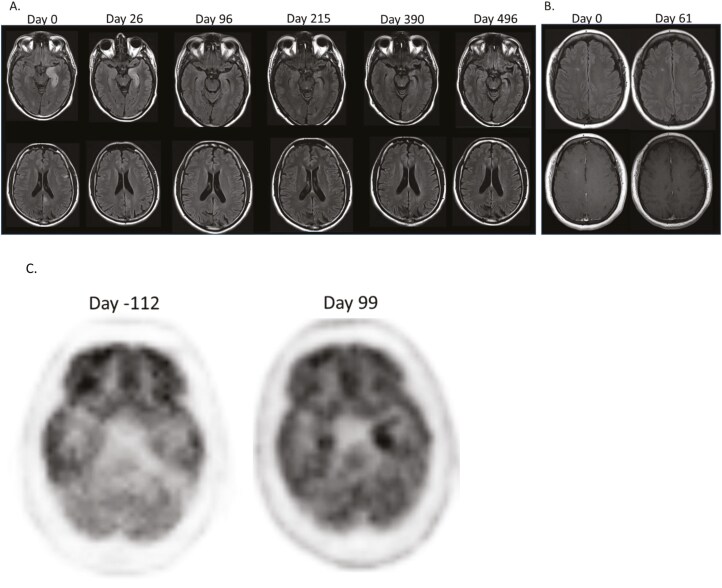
MRI and brain FDG-PET imaging of patients with immune-related encephalitis. (A) T2/FLAIR MRI brain images at presentation and follow up after treatment in a patient with typical imaging findings of autoimmune encephalitis (patient 9). Note T2/FLAIR hyperintensities of the left hippocampus, left frontal lobe, and posterior cingulate. The left hippocampal lesion evolves to medial temporal sclerosis while the left frontal and posterior cingulate lesions resolve. (B) T2 FLAIR and T1-post-gadolinium MRI brain images at presentation and after treatment in patient with lesion of the right corona radiate demonstrating persistence of T2/FLAIR hyperintensity with resolution of gadolinium enhancement on follow-up (patient 6). (C) FDG-PET/CT brain imaging 112 days prior to and 99 days after immunosuppressive treatment initiation for immune-related encephalitis demonstrating hypermetabolism of the left medial temporal lobe that was not evident on the pre-encephalitis FDG-PET performed through the course of oncologic care (patient 9).
